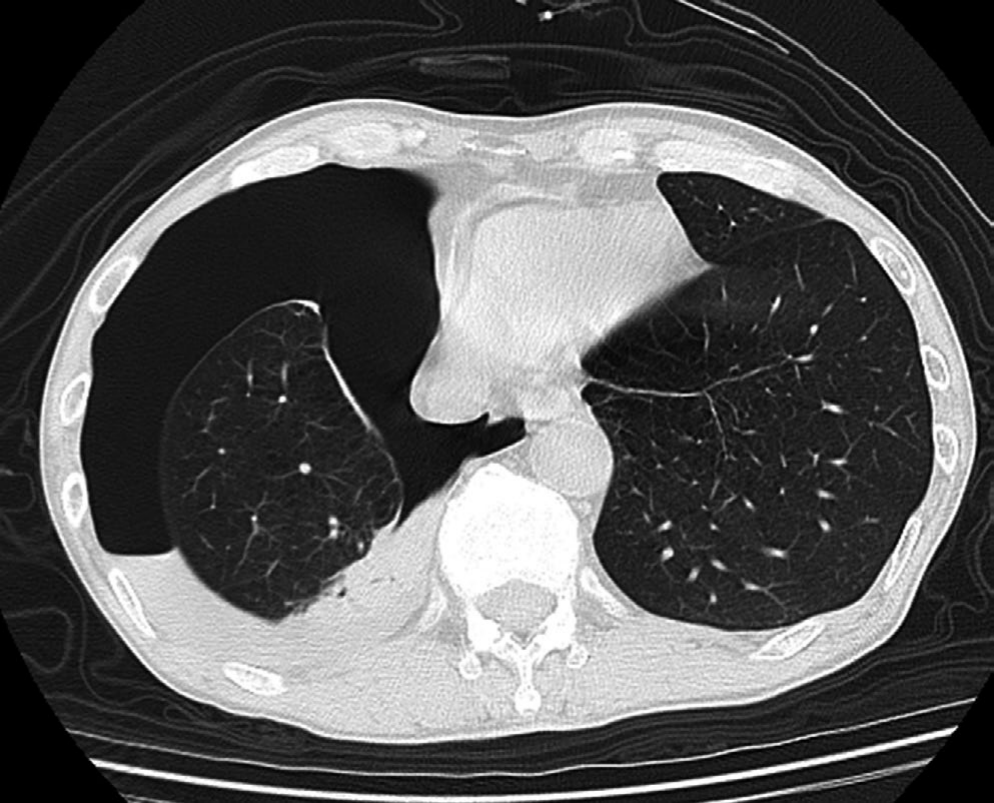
Right pneumothorax revealed by chest computed tomography
